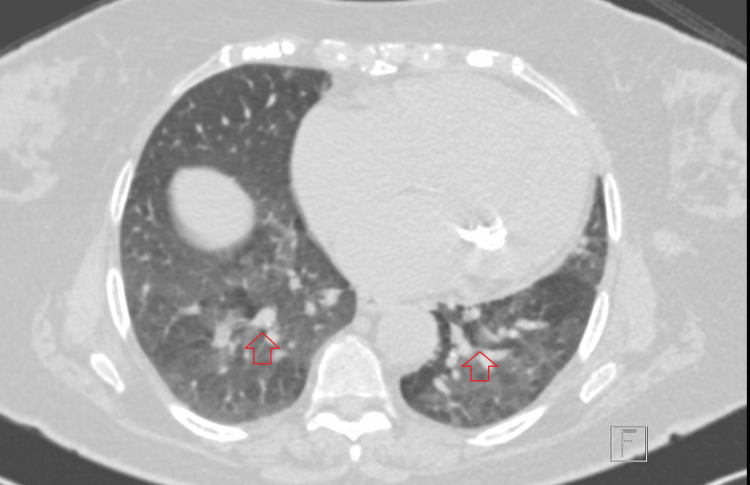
High-resolution CT scan of the chest showing mild interstitial changes and no consolidation
